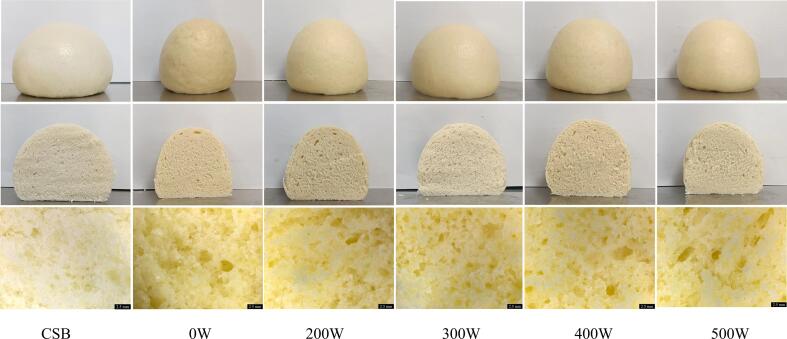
Appearance and crumb microstructure of steamed bread under different ultrasonic power.
